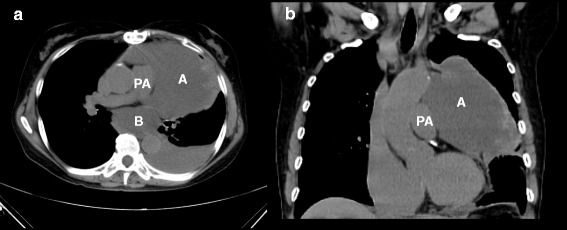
Preoperative computed-tomography images. Computed tomography of a horizontal dislocation and b coronal dislocation showing a large mass in the pericardium anterolateral to the main pulmonary artery (*A*). Horizontal dislocation also showed a mass in the posterior mediastinum posterior to the bronchi (*B*). *PA* pulmonary artery
